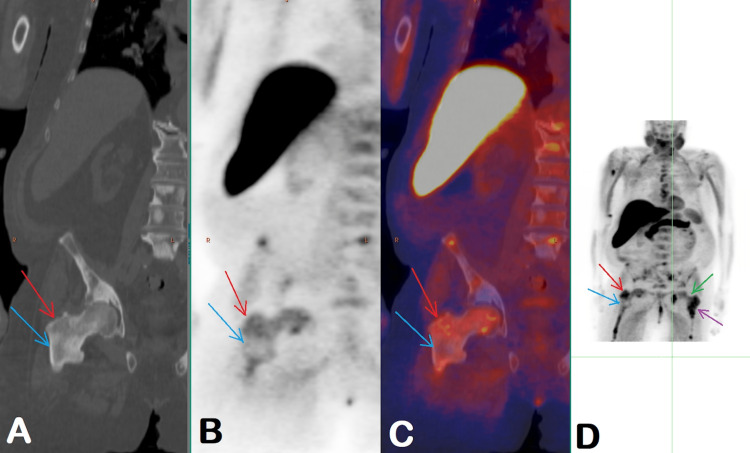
Findings on a fluoride-18 fluciclovine PET-CT scan in June 2022 CT (A), PET (B), fused PET/CT (C), and MIP (D) images show the complete resolution of previously seen abnormal activity in the oldest sclerotic lesion in the intertrochanteric region of the right femur (blue arrows) and decreased intensity of activity in the adjacent sclerotic area (red arrows) with a SUVmax of 4.4 from a previous SUVmax of 5.5 (for reference, background bone marrow activity SUVmax of 2.8). Activity in the previously “hot” lesion in the left hip also decreased (green arrow) with SUVmax of 3.0 from the previous SUVmax of 5.5, but there is new adjacent sclerosis with intense radiotracer activity (purple arrow) with SUVmax 5.5. PET – positron emission tomography, MIP – maximum intensity projection, SUVmax – maximum standardized uptake value
